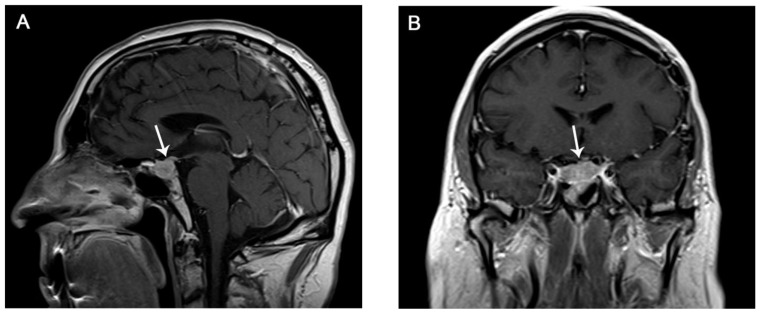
71-year-old male with melanoma, who, after several doses of Ipilimumab, developed fatigue, hallucinations, and laboratory findings of panhypopituitarism. Post-contrast T1-weighted MR sagittal (A) and coronal (B) images revealed enlargement of the pituitary gland and infundibulum with heterogeneous contrast enhancement (arrows in (A,B)). Ipilimumab was discontinued and steroid therapy was started with improvement on the clinical picture. Follow-up MRI was not available.
